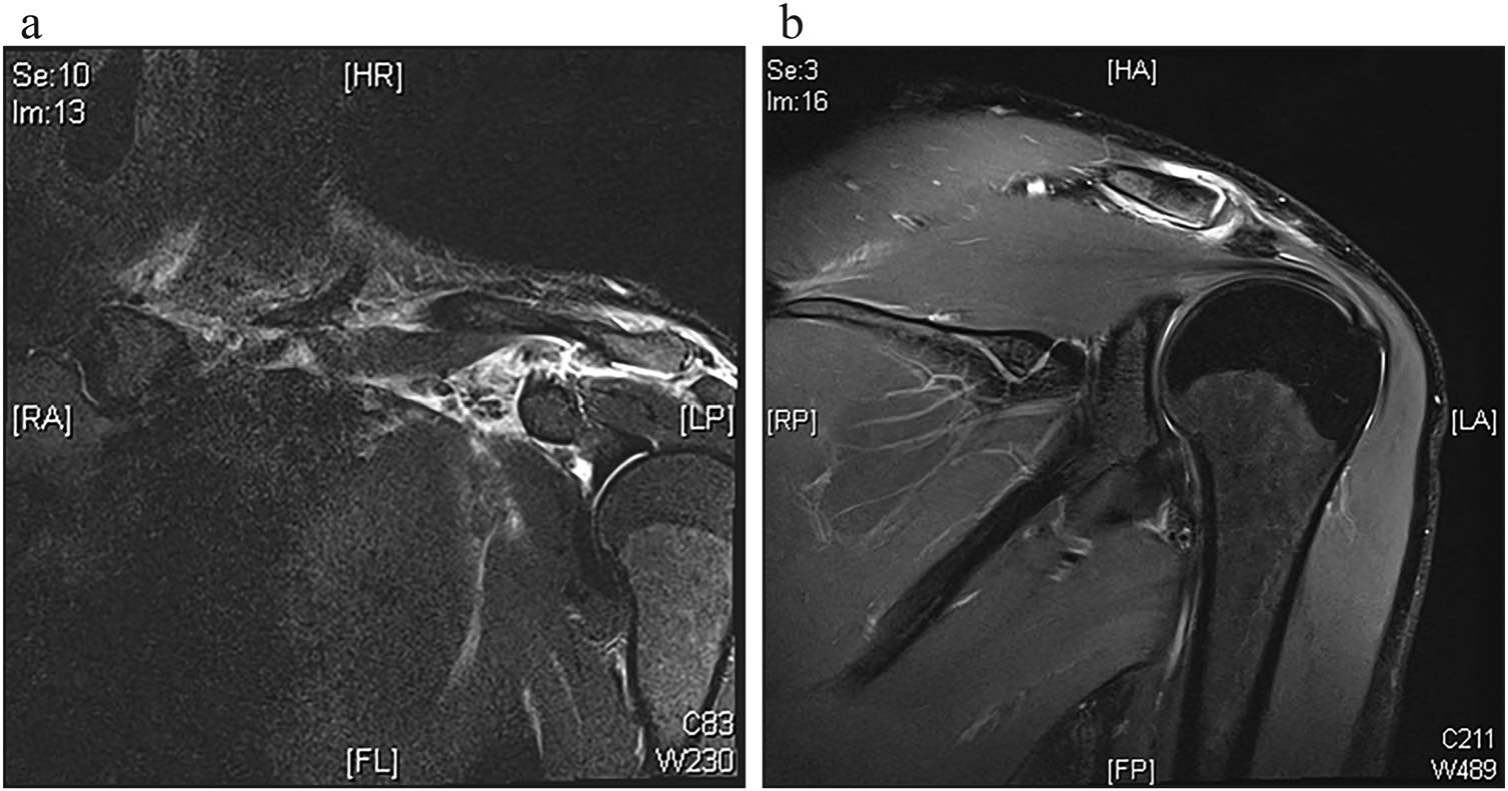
Coronal MRI of the left shoulder region one day after the injury. a A total rupture of the AC and CC ligaments with a concomitant edema surrounding the AC joint, b alongside a ruptured AC capsule is visible
